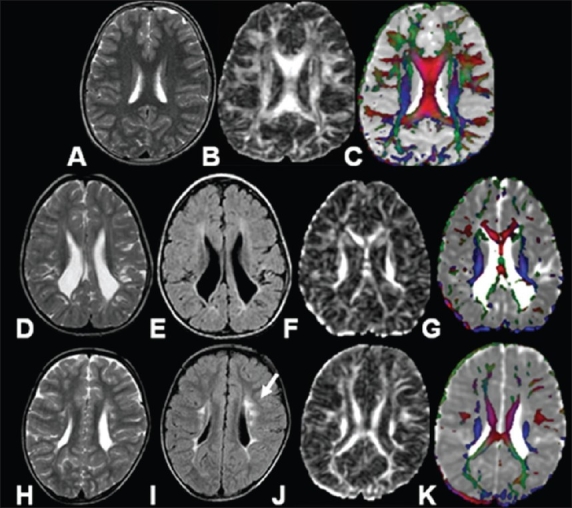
MRI images of an 8-year-old healthy control (A-C). T2W (A), FA map (B), and color-coded FA fused with MD map (C) show normal distribution of white matter. The MRI images of a 9-year-old child (D-G) with a clinical diagnosis of spastic hemiparesis, show nearnormal imaging on T2W (D) and FLAIR (E) sequences. The FA map (F) shows decreased FA values in the periventricular white matter region as compared to the control (C) that is clearer on the color-coded FA fused with MD map (G). MRI images of a 12-year-old child (H-K) with a clinical diagnosis of spastic hemiparesis. T2W (H) and FLAIR (I) images show hyperintense lesions (arrow) in the periventricular white matter. The FA map (J) shows decreased intensity in the periventricular white matter that is clearer on the color-coded FA fused with MD map (K)
